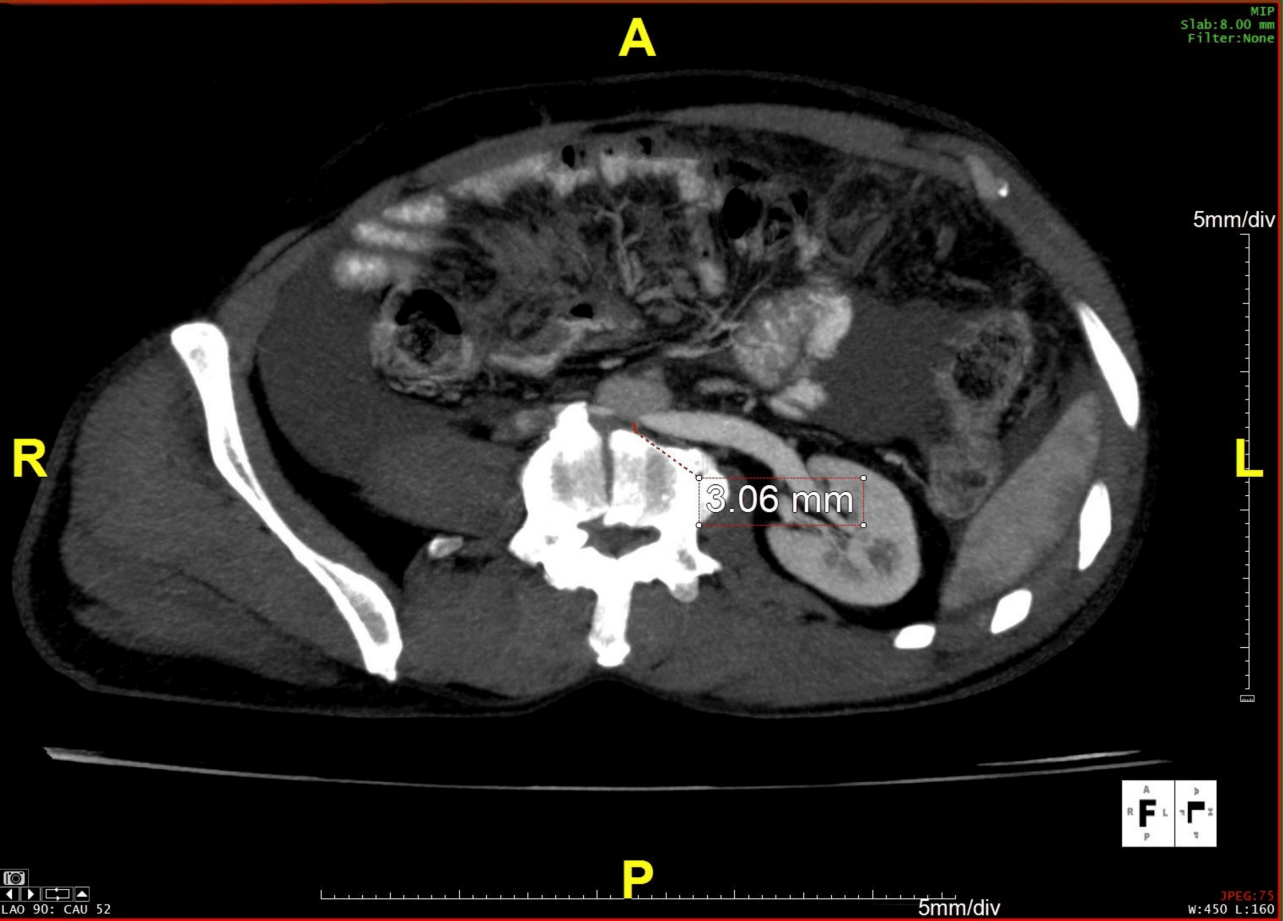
Axial section on contrast-enhanced CT with maximum intensity projection, showing an aorto-vertebral distance of 3.06 mm
